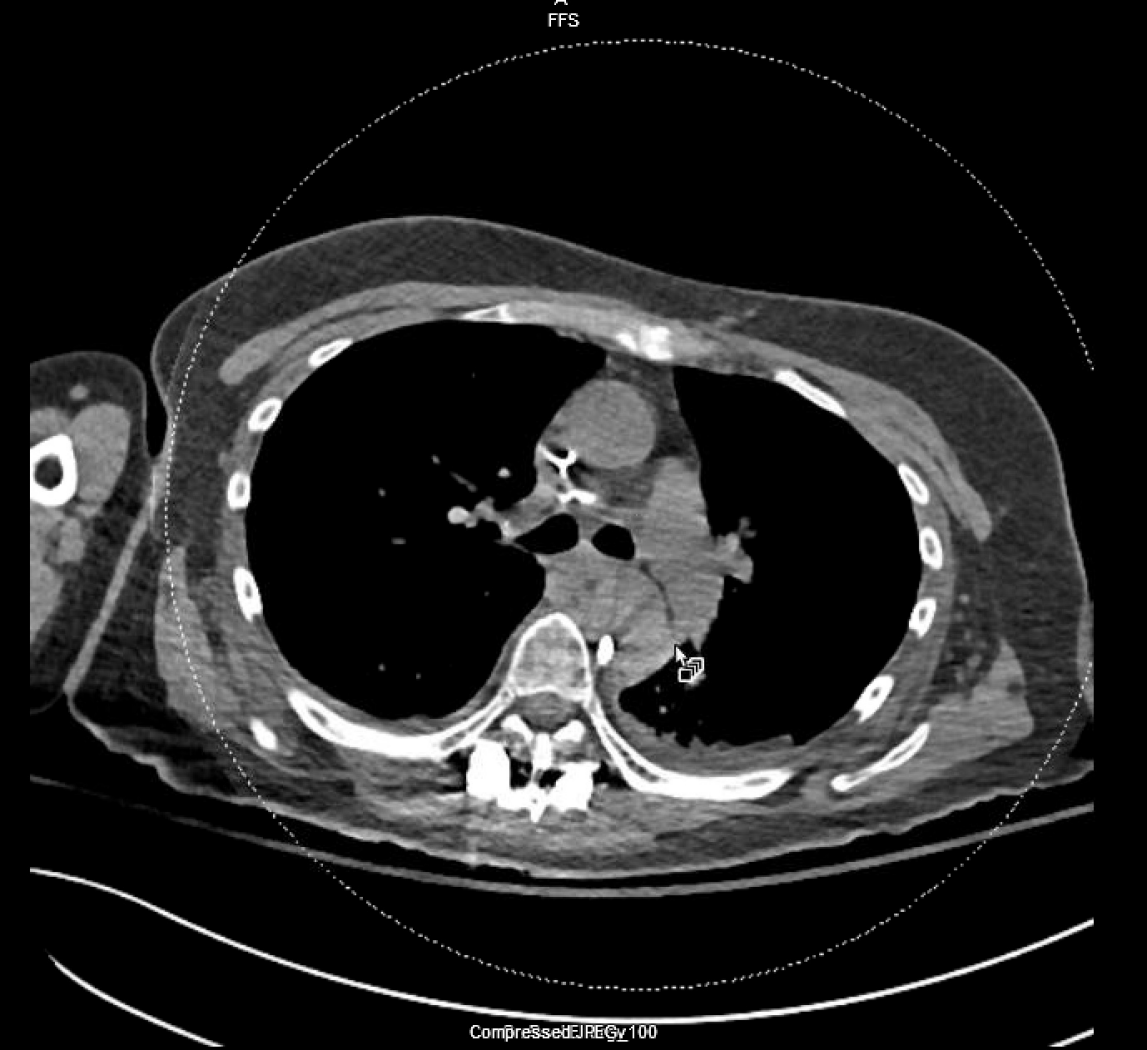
Computed tomography (CT) scan of the chest An aortic intramural hematoma is demonstrated with screw abutting the proximal descending thoracic aorta.
